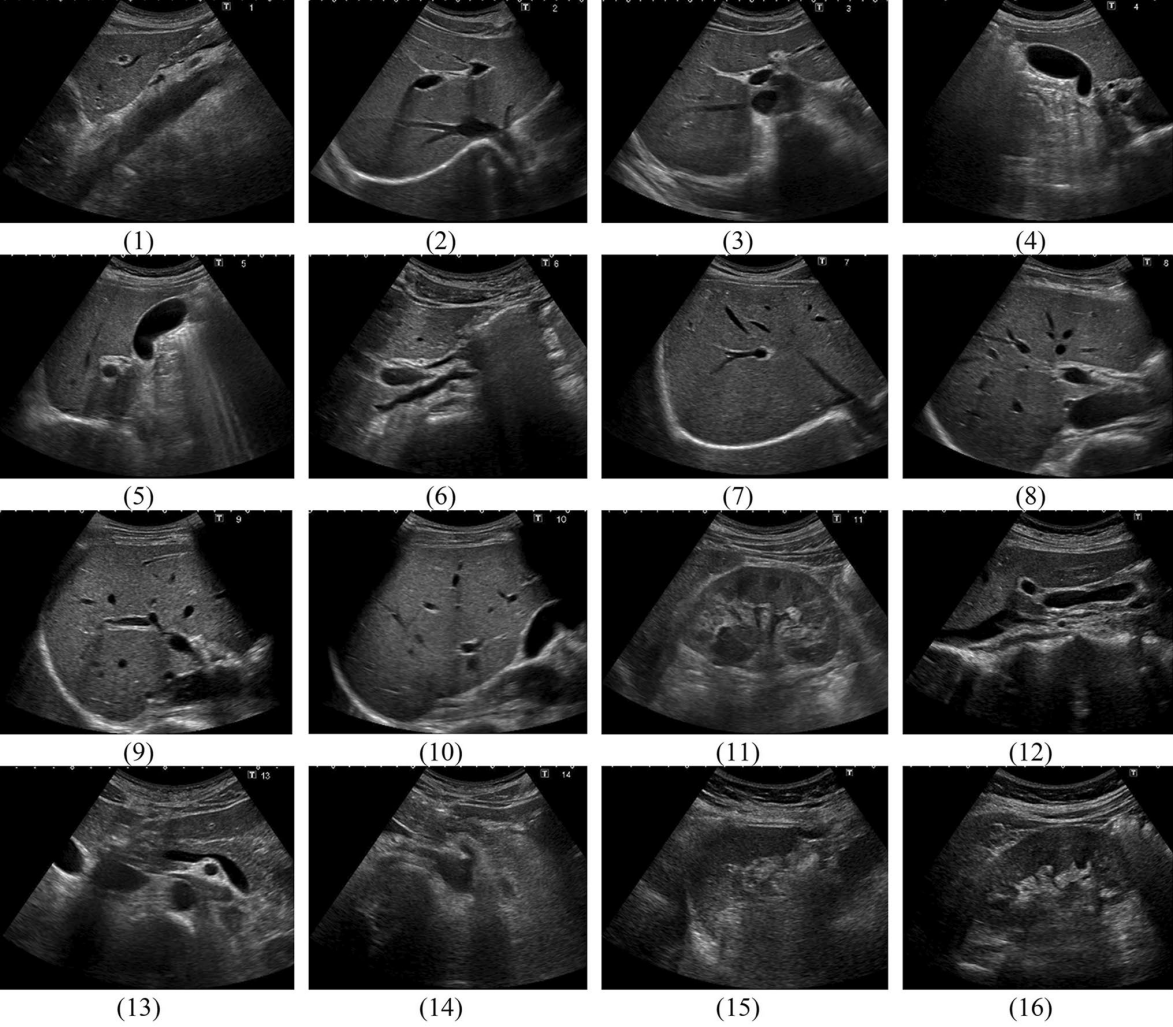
Example of the 16 upper abdominal ultrasound cross sections: 1. Epigastric sagittal (liver/aorta), 2. Epigastric horizontal (hepatic vein), 3. Right Epigastric oblique (horizontal portal vein), 4. Right Subcostal (gallbladder), 5. Right hypochondrium vertical (gallbladder), 6. Right hypochondrium vertical (bile duct), 7. Right subcostal (liver), 8. Right intercostal (liver), 9. Right intercostal (liver), 10. Right intercostal (liver), 11. Right intercostal (kidney), 12. Epigastric vertical (bile duct/pancreas), 13. Epigastric horizontal (pancreas), 14. Epigastric oblique (Pancreas), 15. Spleen, 16. Left intercostal (kidney)
